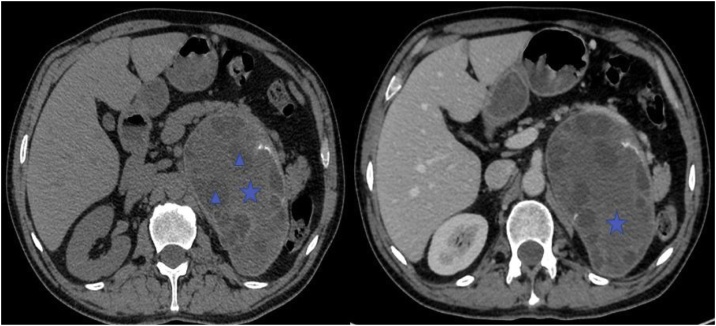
Axial unenhanced and contrast-enhanced axial CT showed a cystic echinococcus 3b of the WHO classification: unilocular cystic well defined cystic mass with daughter cysts  inside a mucinous or solid cyst matrix  (high density region). No significant enhancement has been detected in any part of the cyst.
